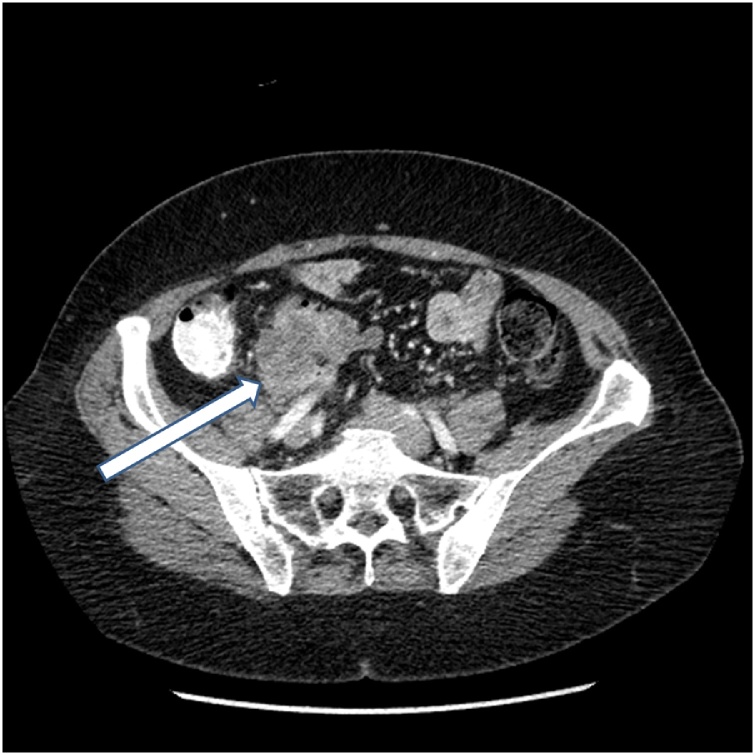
CT slice through a primary caecal adenocarcinoma. The greatest dimension of the mass was 8 cm. The arrow indicates the cancer extension into the retroperitoneum. Colonoscopic biopsy showed a poorly differentiated cancer.
